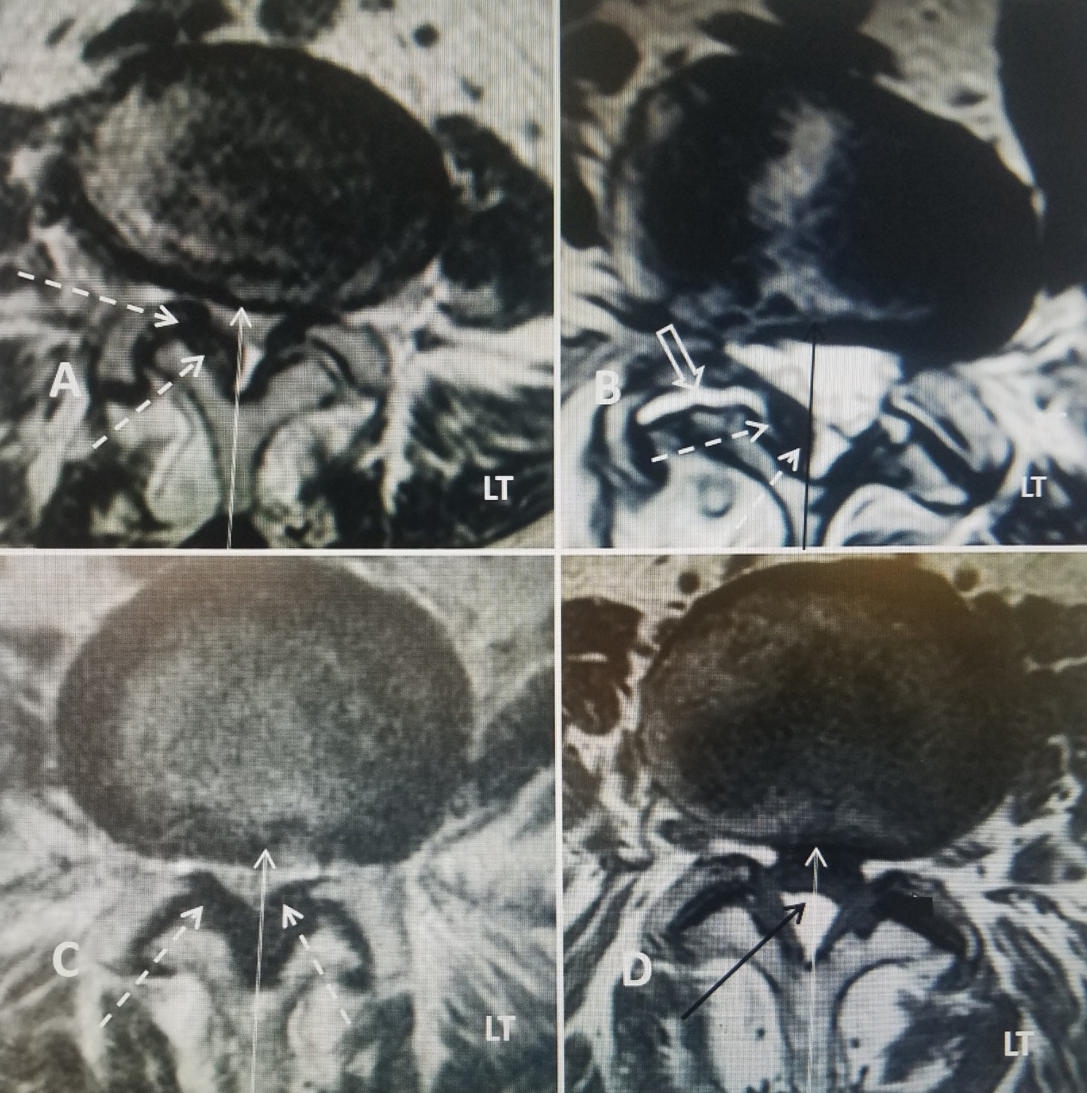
Four cases showing the axial T2 MRI at L4-5 showing different anatomic locations and severity of the stenosis from central stenosis to lateral recess stenosis. Left is marked (LT). When correlated with clinical symptoms and findings, different methods of correcting the lumbar stenosis may be indicated. A: Axial T2 MRI showing bilateral but more marked right lateral recess stenosis due to ligamentous hypertophy (dashed white arrow). The midline is marked (thin white line). B: Axial T2 MRI demonstrating right asymmetric facet hypertrophy with an enlarged right facet joint and unilateral lateral recess stenosis (dashed white arrow). There is a high T2 signal in the facet joint (open white arrow) indicating facet joint fluid, a sign of possible instability. There is additional ligamentous hypertrophy only on the right side indicated by thick black MRI signal under the lamina adjacent to the facet joint (dashed white arrows). The enlarged facet joint is encroaching on the midline (solid thin black arrow) but the left side is normal. C: Axial T2 MRI with thick symmetric ligamentous hypertrophy causing severe canal stenosis (dashed white arrows). The ligamentous hypertrophy (solid white arrows) completely encroaches up to the midline (thin white line). D: Axial T1 MRI showing bilateral symmetric facet hypertrophy. The midline is marked (thin solid white line) and there is a facet fluid cyst causing dural compression (solid black arrow).
